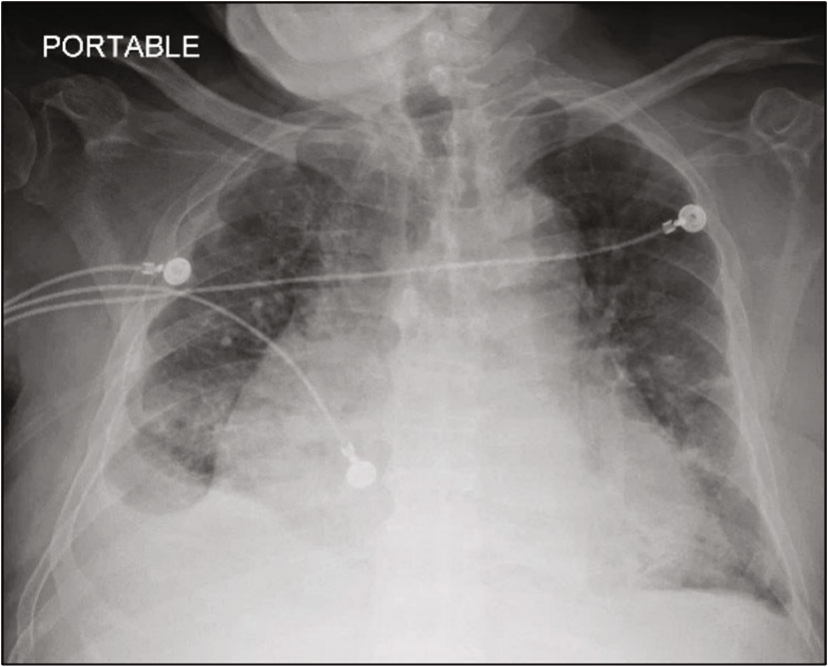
Chest X-ray showing pulmonary congestion, bilateral pleural effusion, cardiomegaly, and possible consolidation in the right lower lobe.
